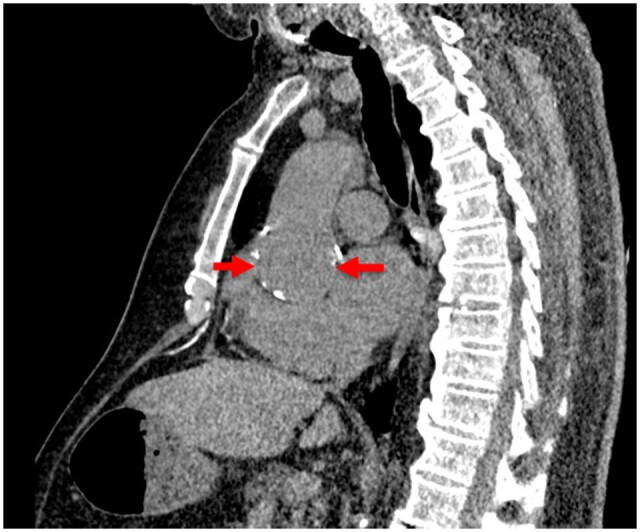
Sagittal missed findings for aortic dilatation (arrows) on a non-contrast CT chest.
